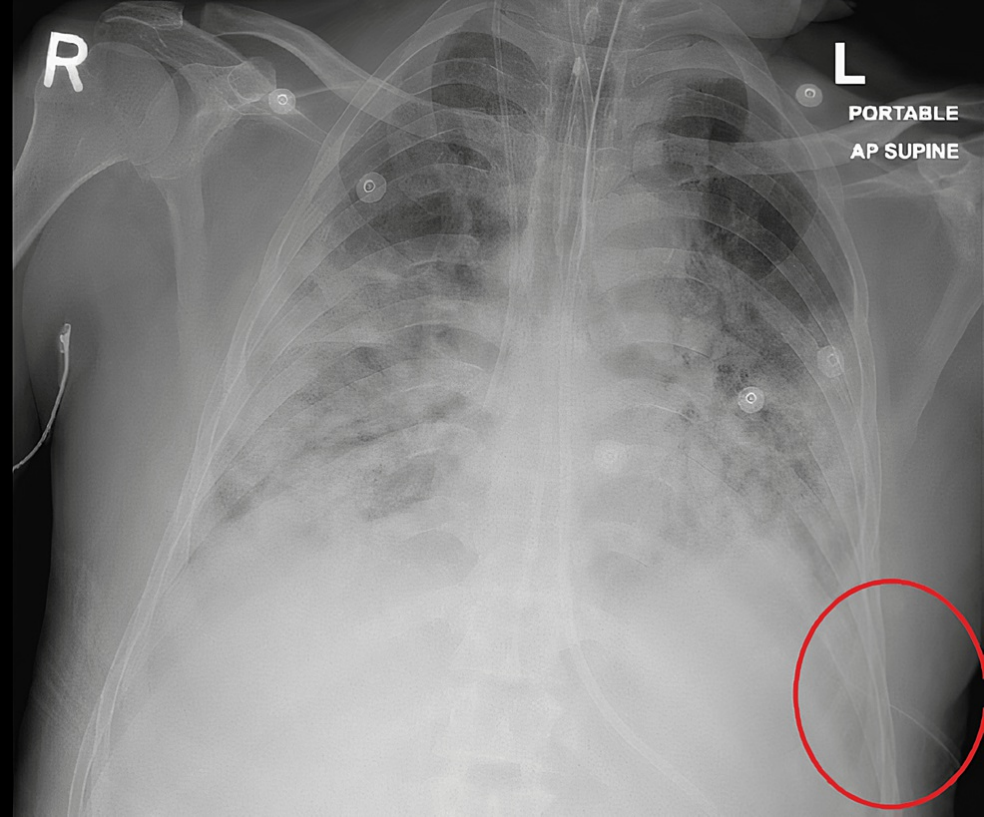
Chest X-ray Post thoracostomy tube insertion
